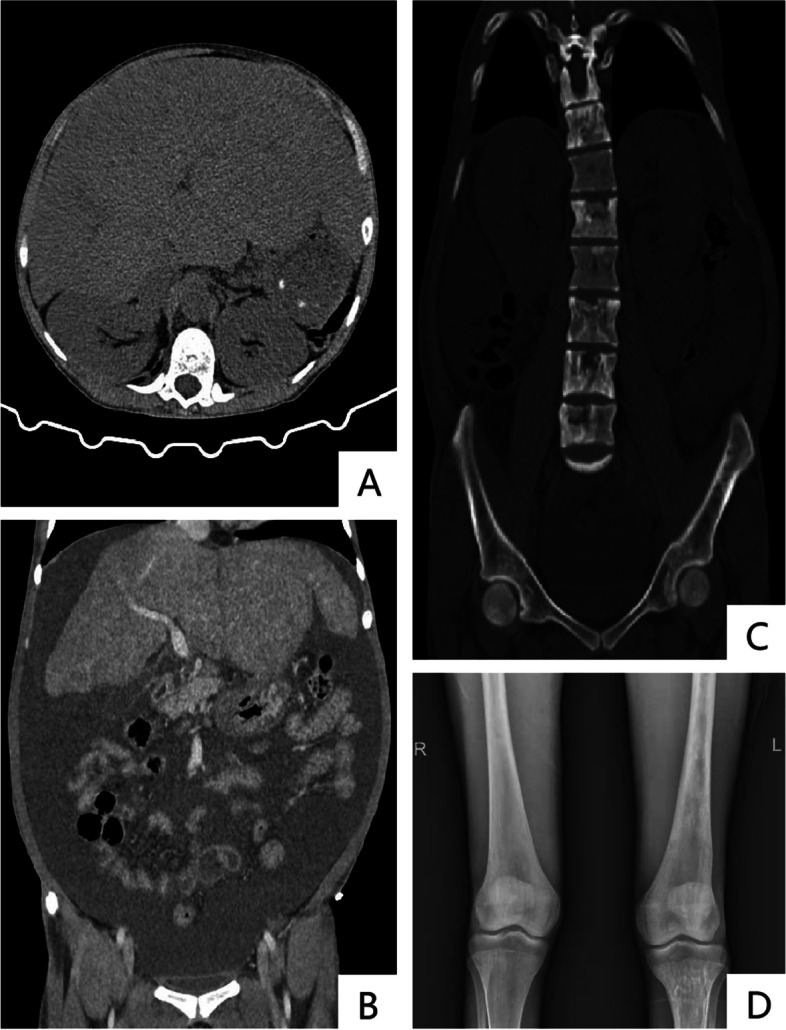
A CT scan showed diffusely enlarged liver. B CT scan showed abundant ascites. C CT scan showed bone destruction in multiple thoracolumbosacral vertebrae especially in sacrum and left ilium. D Femoral radiographs showed decreased bone density and symmetrical widening of middle and lower segments of both femurs, which looked like the "beer bottle shape" changed. CT=Computed tomography
